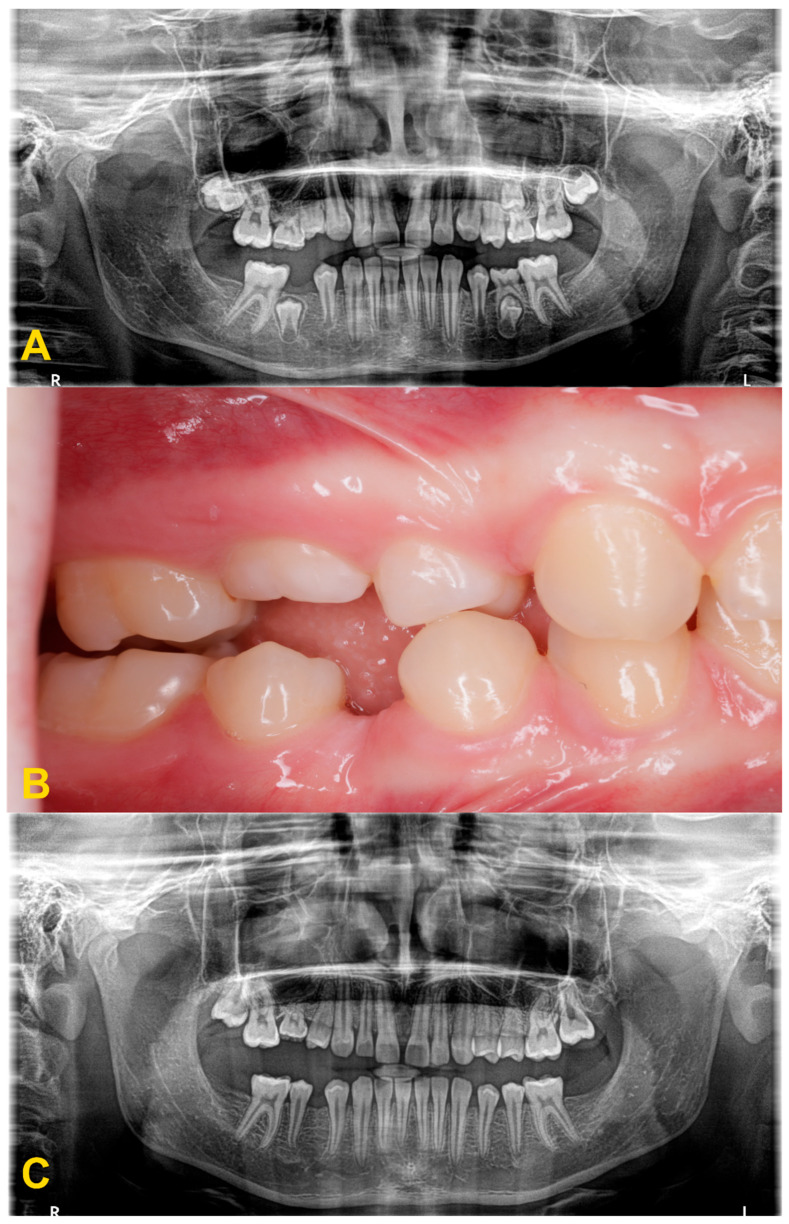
Progression of infraocclusion in tooth no. 55 in the absence of a permanent successor. Extraction is recommended due to the possible progression of the bone defect and inclination of adjacent teeth. Spontaneous exfoliation of tooth no. 75 with infraocclusion and self-correction of second premolar’s distoinclination. Missing teeth nos. 37 and 47. Failure of eruption of teeth nos. 17 and 27, surgical exposure is indicated. (A) panoramic X-ray, (B) intraoral photography, and (C) panoramic X-ray with 4-year follow-up.
